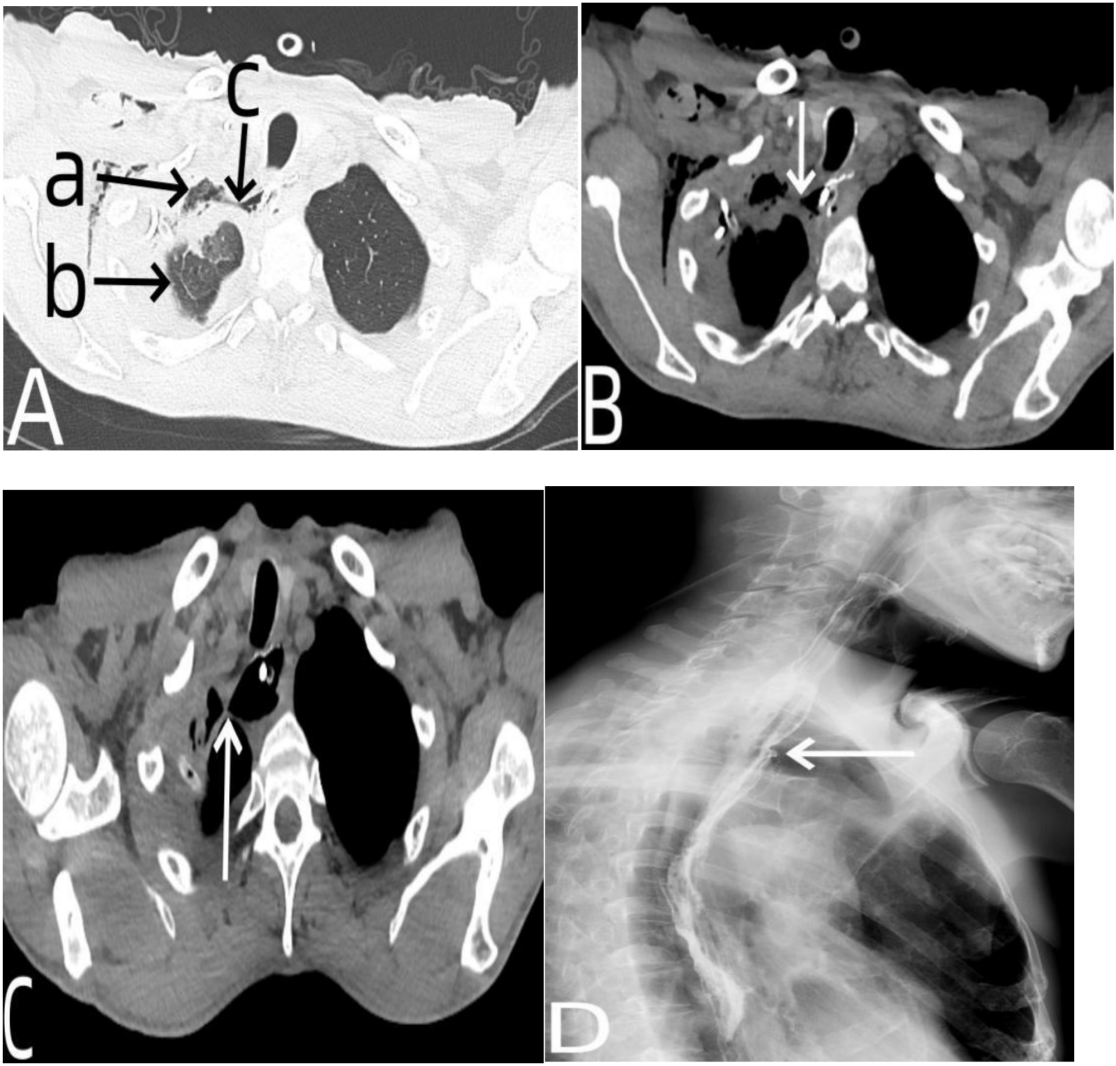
(A, B) showed the chest CT scan on postoperative day 9. In (A) which was the lung window, the arrow a indicated that the residual cavity in the thoracic cavity had nearly disappeared, the arrow b showed that the right lung had almost fully re-expanded, and the arrow c indicated that the anastomotic fistula had been closed. In (B) which was the mediastinal window, the arrow showed that the anastomotic fistula had been closed. (C) showed the chest CT on postoperative day 18, with the arrow indicating that the anastomotic fistula had completely closed. (D) showed the contrast esophagography on postoperative day 18, with the arrow marking the original site of the anastomotic fistula, where no contrast agent leakage was observed.
